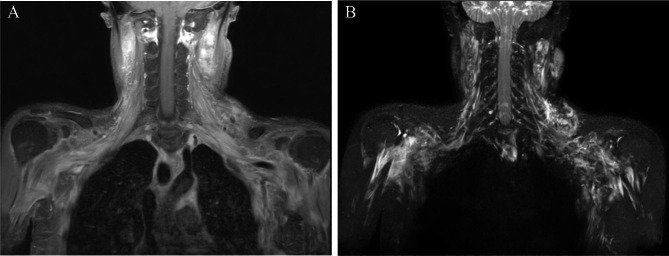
Brachial plexus magnetic resonance images show thickening with a diffuse enhancement of the entire segments of both brachial plexuses. (A) T1- and (B) T2-weighted magnetic resonance images of the brachial plexuses show thickening with a diffuse enhancement of the entire segments of both brachial plexuses
